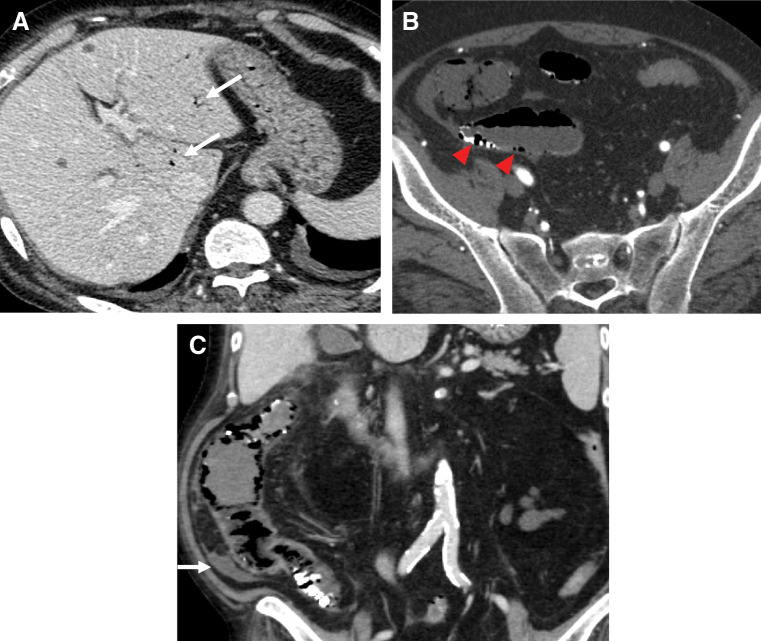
Contrast-enhanced CT findings. (A) Axial image showing the presence of hepatic portal venous gas (white arrows). (B) Axial image showing thinned intestinal wall and pneumatosis intestinalis of the small intestine (red arrowheads). (C) Coronal image showing thinned and poorly contrasted intestinal wall and bloody ascites (white arrow).
